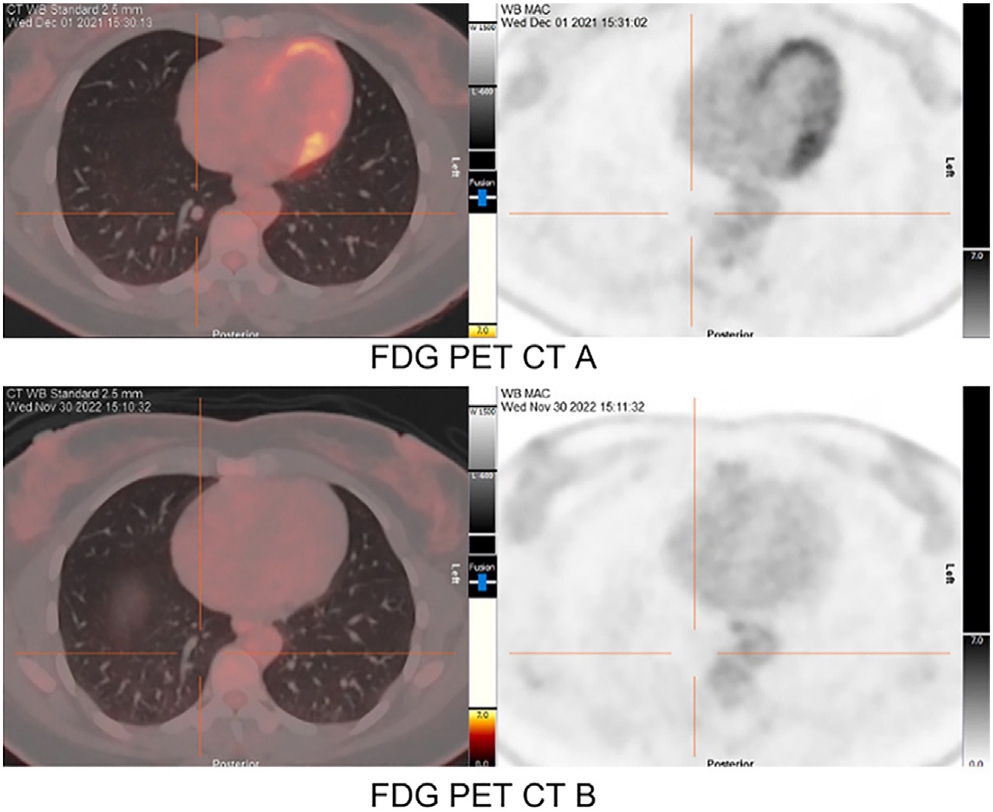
Case 2 FDG PET-CTs (left panels) and maximum intensity projection (MIP) (right panels) obtained before and after larotrectinib treatment for target lesion 1. Pre-larotrectinib therapy FDG PET conducted in December 2021 is represented by FDG PET A. In comparison, FDG PET B was obtained post larotrectinib therapy in November 2022, demonstrating a reduction in size and decreased FDG-PET avidity of the target lesion.
